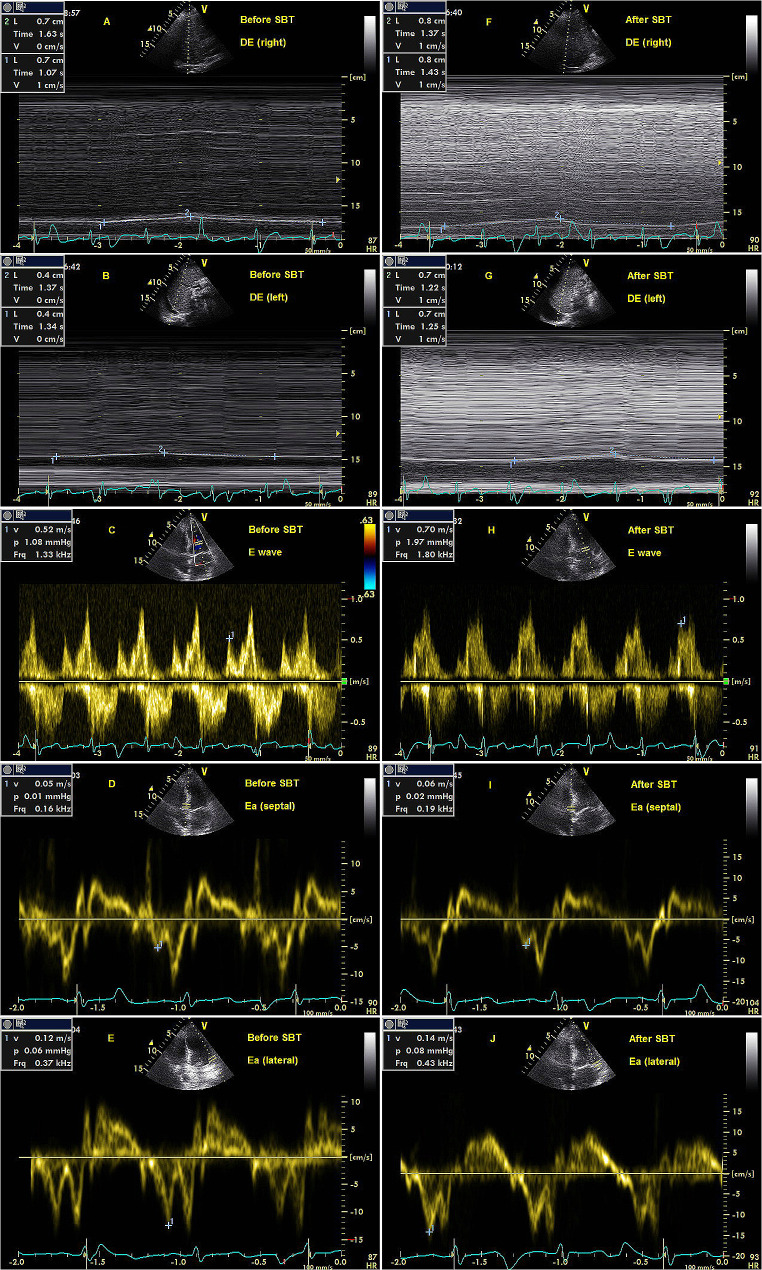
E/Ea and DE measurements during a SBT in a patient of re-intubation within 1 week. A, B, C, D, E represented the measurements before SBT, and F, G, H, I, J represented the measurements after SBT. (A) DE (right) was 7 mm. (B) DE (left) was 4 mm. (C) E was 52 cm/s. (D) Ea (septal) was 5 cm/s. (E) Ea (lateral) was 12 cm/s. (F) DE (right) was 8 mm. (G) DE (left) was 7 mm. (H) E was 70 cm/s. (I) Ea (septal) was 6 cm/s. (J) Ea (lateral) was 14 cm/s. Before SBT, the resultant DE (average) was 5.5 mm, and E/Ea (average) was 6.1. After SBT, the resultant DE (average) was 7.5 mm, and E/Ea (average) was 7. Respiratory failure did not occur within 48 h after extubation. However, the patient required re-intubation at the third day due to severe hypoxemia. DE: diaphragmatic excursion; E/Ea: the ratio of mitral Doppler inflow velocity (E) to annular tissue Doppler wave velocity (Ea); SBT: spontaneous breathing trial
